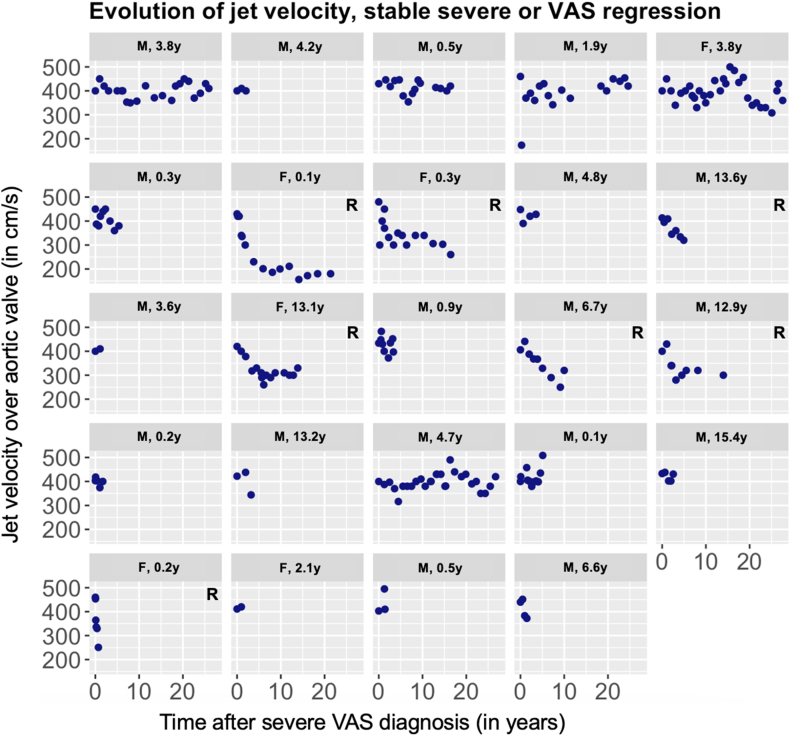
Serial Doppler jet velocities for the 24 children with a decrease or stabilization of their gradient without intervention. The Y-axis represents the peak jet velocity (in cm/s) measured over the aortic valve by use of Doppler echocardiography; the X-axis represents time (in years). R = gradient Regression.
